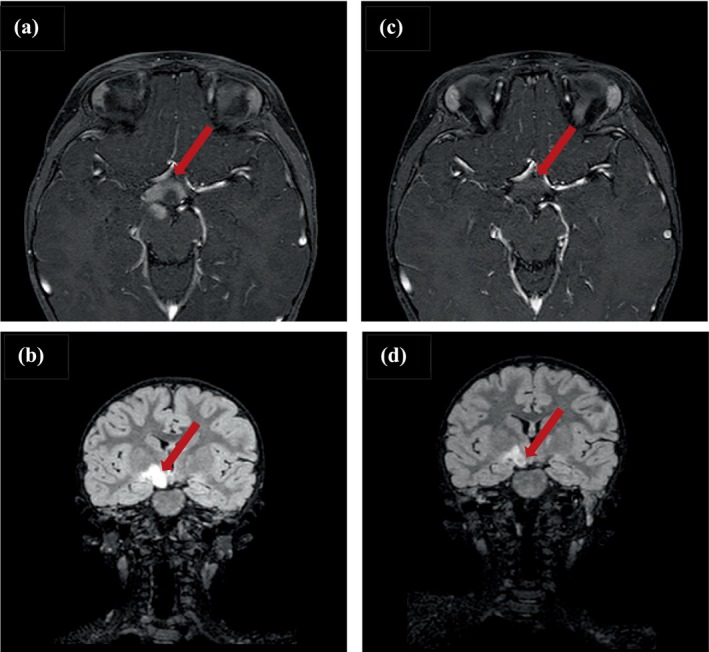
Pre‐therapy MRI (a and b) shows the glioma with contrast enhancement (a) and T2‐FLAIR alterations (b). Post‐selumetinib MRI (c and d) at 4 months displays an almost complete absence of contrast enhancement (c) and a reduction in glioma size (d).
